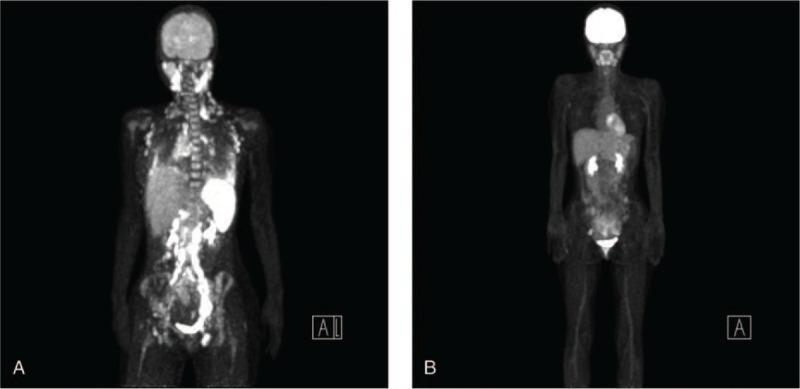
(A). PET/CT scan on day 16 of admission shows FDG uptake in systemic lymph nodes, lungs, liver, spleen, and bones. (B). PET/CT scan on day 43 of crizotinib treatment. No abnormal FDG uptake is shown.
